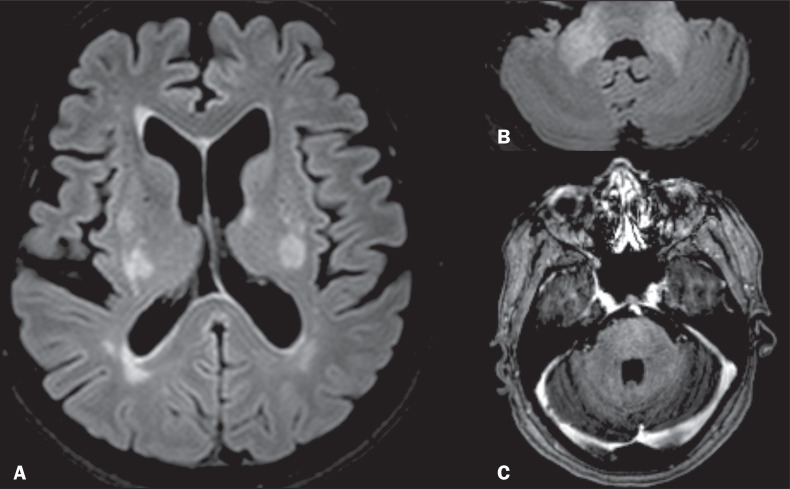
ADEM. A: Axial FLAIR sequence demonstrating supratentorial
